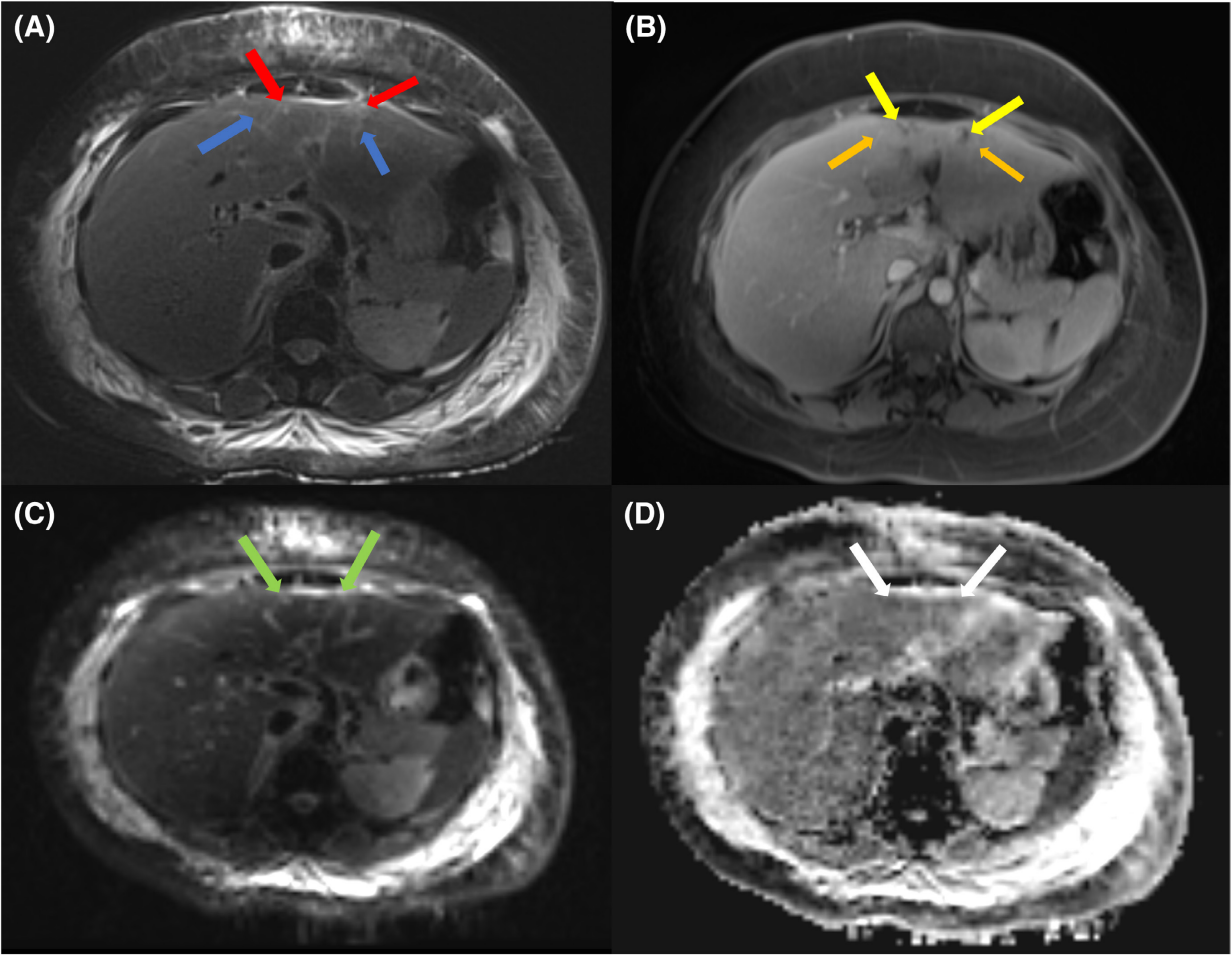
MRI Abdomen with intravenous contrast (A) T2 blade fat sat, (B) T1 post contrast venous phase, (C) DWI and (D) ADC, demonstrating T2 subcapsular linear high signal intensities in the left hepatic lobe (Blue arrows) which show heterogenous enhancement (Orange arrows). Multiple small T2 bright clusters (Red arrows) are seen, which are non‐enhancing on T1 post contrast (Yellow arrows). The small clusters of foci show high signal on DWI (Green arrows) and low signal on ADC (White arrows), suggestive of diffusion restriction.
